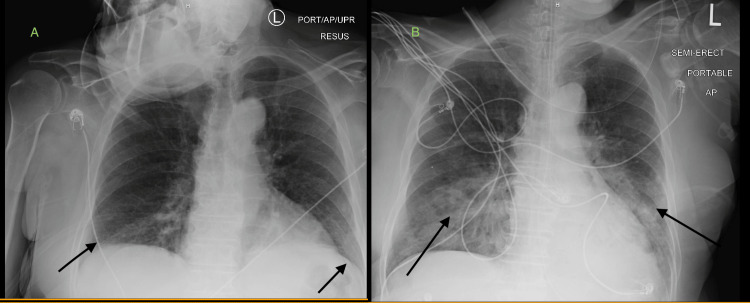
Chest X-ray images (1A) Black arrows show patchy opacity within the lung bases; (1B) Black arrows show bilateral mid and lower lung field airspace opacities.
